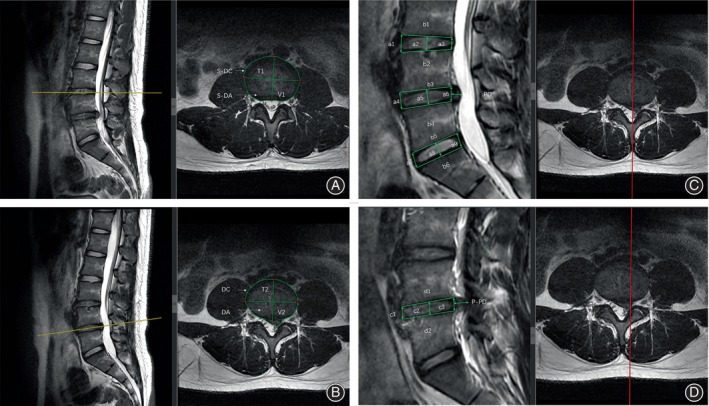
Measurement of MRI parameters. (S‐ = superior segment, P‐ = parasagittal plane, I‐ = inferior segment). (A) superior segment: The vertical diameter (VD, mm), the transverse diameter (TD, mm), the disc circumference (DC, cm), and the disc area (DA, cm^2^). S‐VD=V1, S‐TD = T1, S‐AR = V1/T1. (B) operative segment: The vertical diameter (VD, mm), the transverse diameter (TD, mm), the disc circumference (DC, cm), and the disc area (DA, cm2). VD = V2, TD = T2, AR = V2/T2. (C) Median sagittal plane: The disc height (DH, mm), the disc height index (DHI), the protrusion distance (PD, mm), S‐DH = (a1 + a2 + a3)/3, DH = (a4 + a5 + a6)/3, I‐DH = (a7 + a8 + a9)/3, S‐DHI = (a1 + a3)/(b1 + b2), DHI = (a4 + a6)/(b3 + b4), I‐DHI = (a7 + a9)/(b5 + b6). (D) parasagittal plane: The disc height (DH, mm), the disc height index (DHI), the parasagittal protrusion distance (PD, mm), P‐DH = (c1 + c2 + c3)/3, P‐DHI = (c1 + c3)/(d1 + d2).
